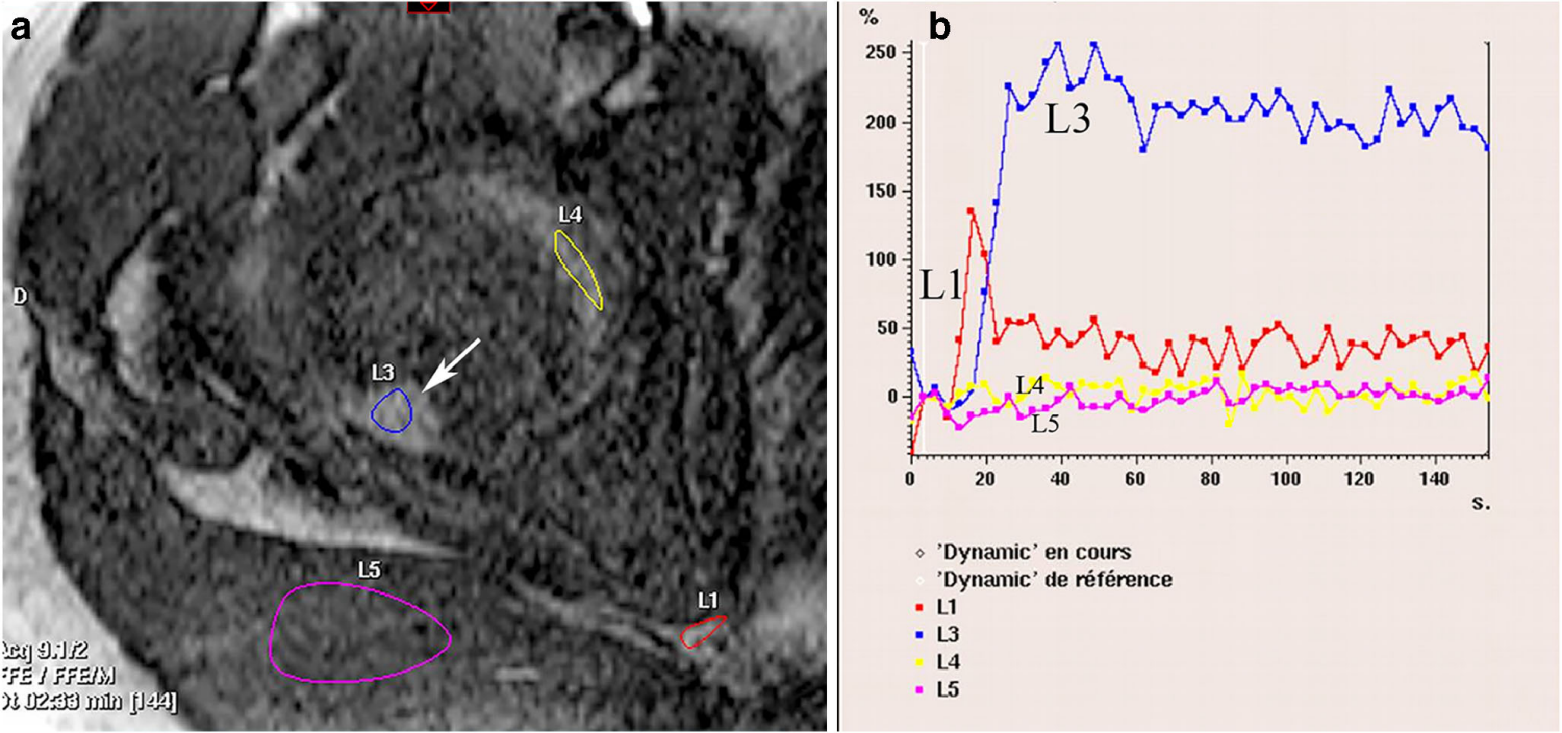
DCE-MRI of an OO. Axial post-contrast gradient-echo T1-weighted MR image of a femoral head OO (arrow in a) (same case as Fig. 4). Signal enhancement curves (b) show that the enhancement of the OO (L3) starts 3 s after that of the artery (L1) with a similar slope. Limited enhancement is measured in the joint cavity (L4) and in a muscle (L5)
